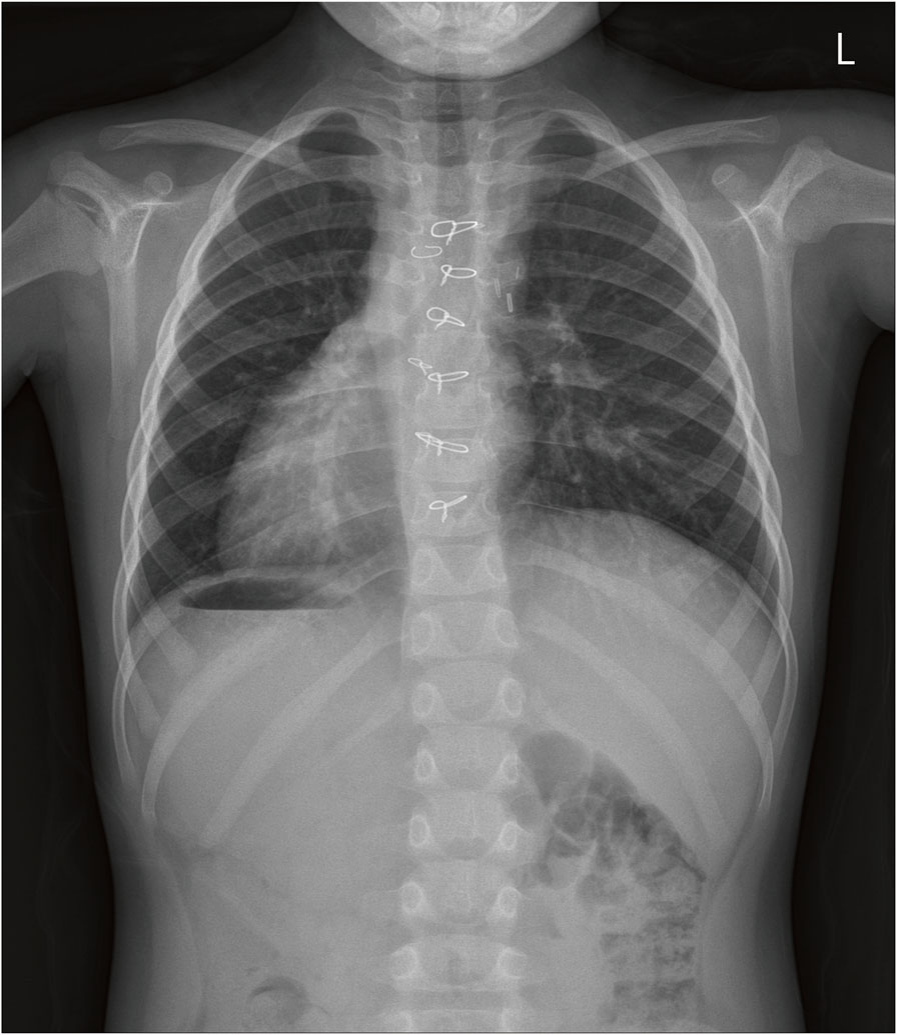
Preoperative chest radiography showing situs inversus totalis with dextrocardia
